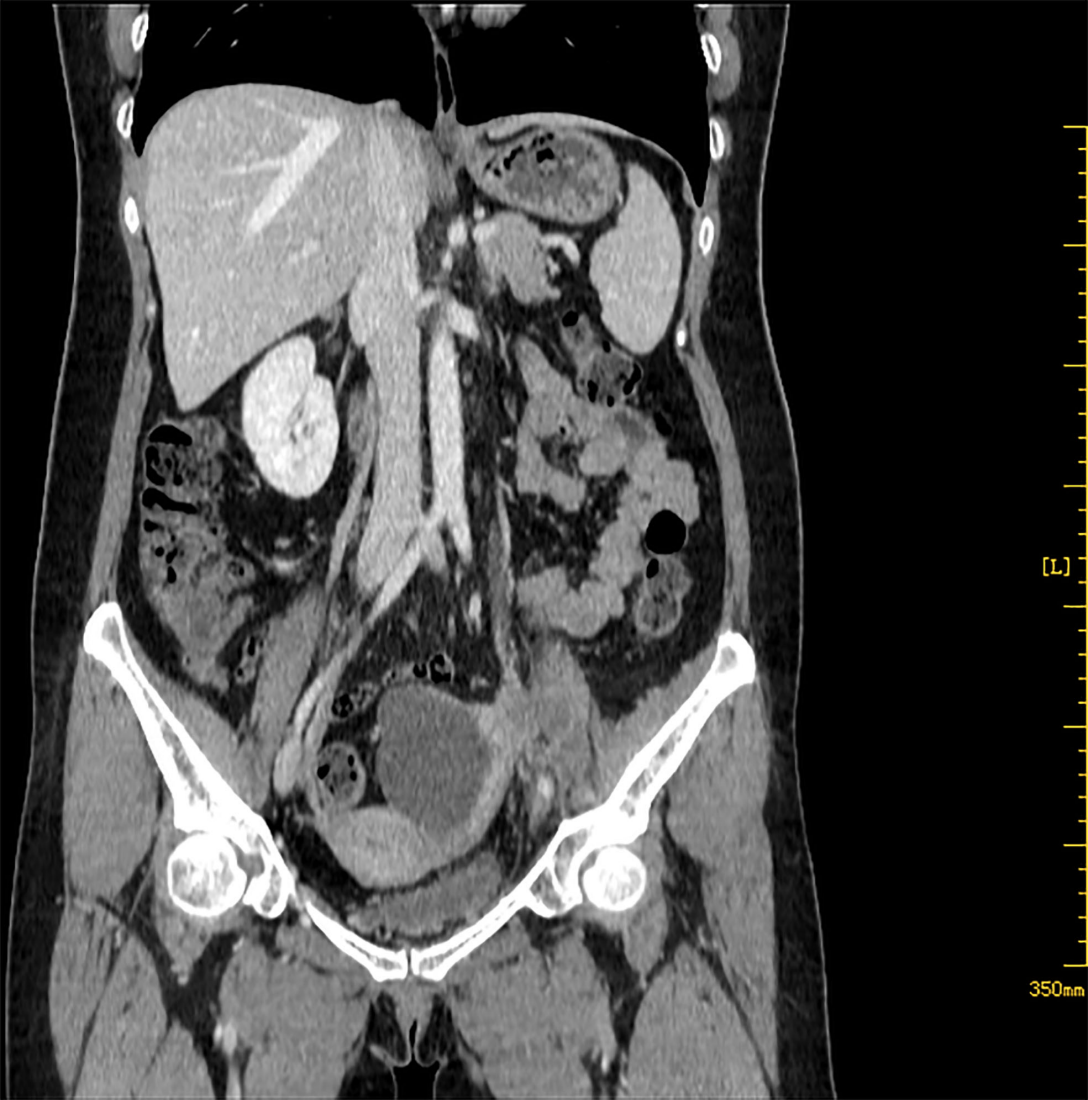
Abdominal CT shows a 7.1 × 4.3 × 5.4 cm septal cystic, solid mass was detected on the left adnexal, and the solid components were enhanced.
